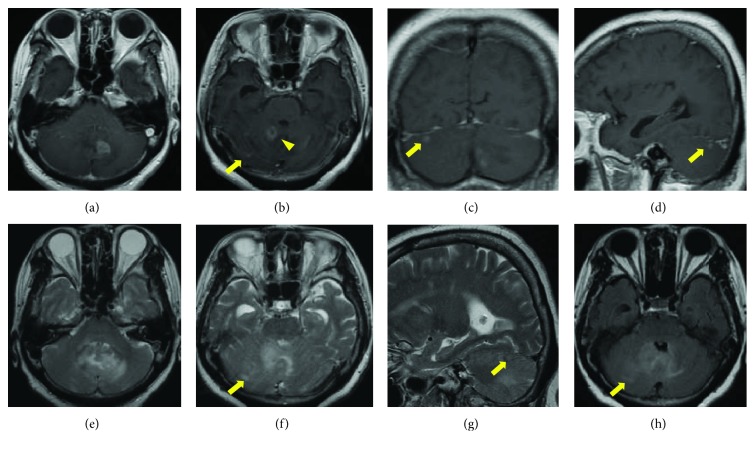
Magnetic resonance images (MRIs) of the patient before biopsy. (a-d) Gd-enhanced T1-weighted images (Gd-T1WI) showing three separate lesions in the cerebellum: the lesion in the left cerebellum near the vermis with relatively regular enhancement (a), the lesion with the similar size in the right cerebellum with ring enhancement (b, arrowhead), and the tiny lesion in right cerebellum located far from two lesions (b, c, d, arrow). T2-weighted images (T2WI) showing peritumoral edema around two lesions (e, f, g), but no edema around the tiny lesion (f, arrow). FLAIR showing no clear connection of the tiny lesion to other two lesions (h, arrow).
